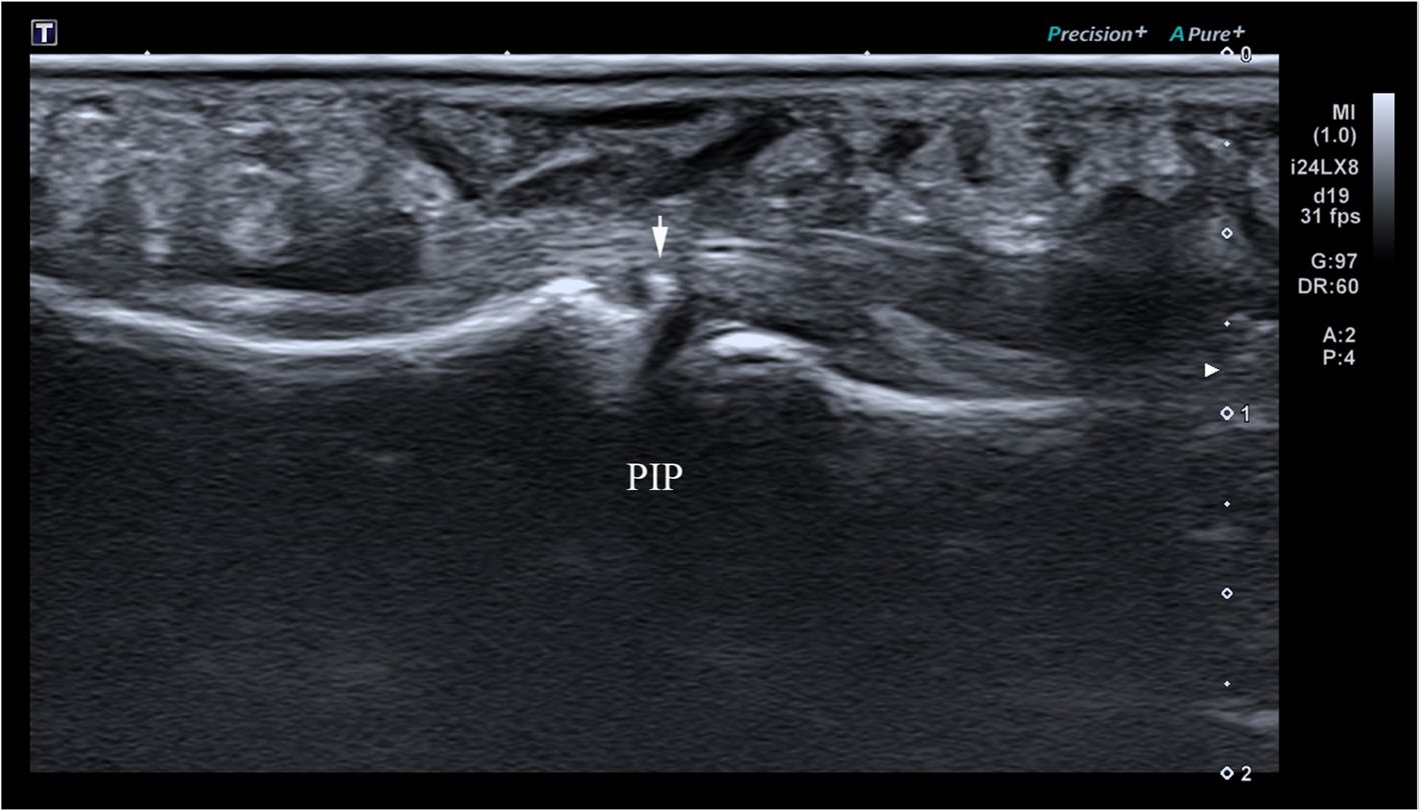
Type A (avulsion fracture without volar plate rupture). High frequency ultrasonography image of type A volar plate injury of a 21-year-old female in longitudinal plane. The arrow shows the avulsion bony fragment of the middle phalangeal base. PIP: proximal interphalangeal joint
